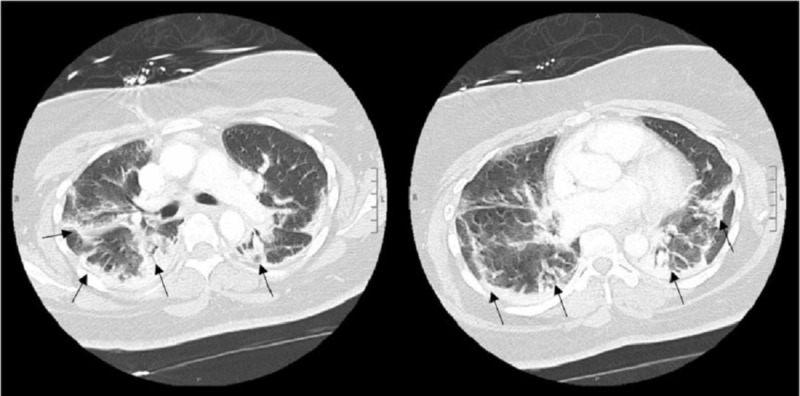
Chest CT on hospital day 2 showing peripheral patchy airspace opacities.
